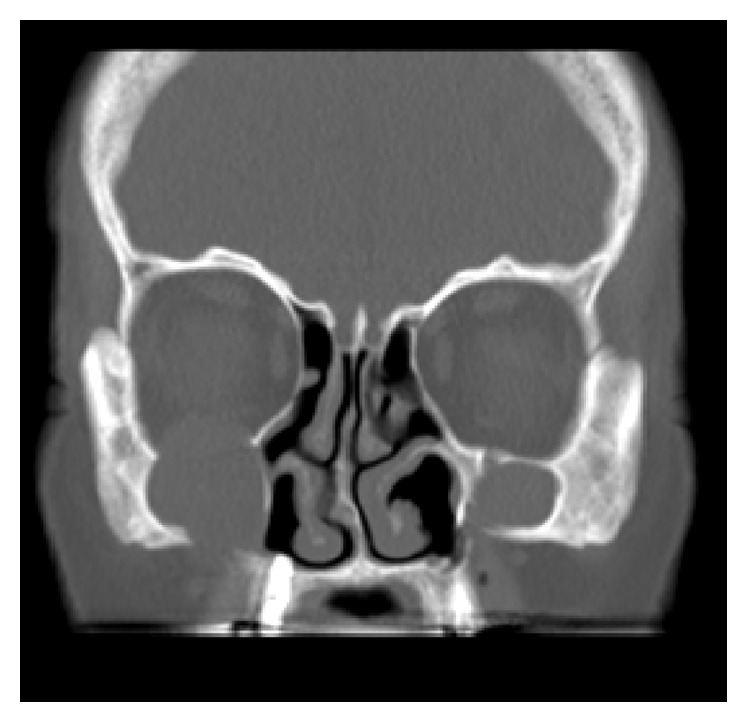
CT: coronal view showing osseous defects in the nasal part of the right sinus and the orbital floor.
